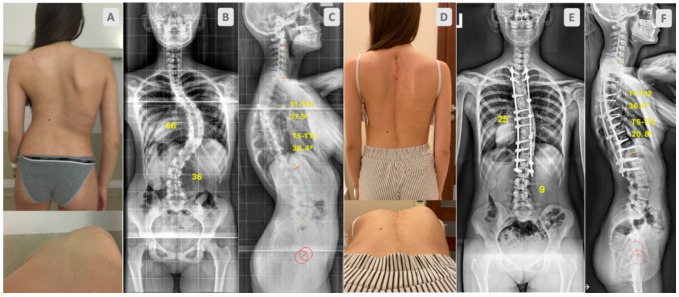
Sixteen-year-old girl with adolescent idiopathic scoliosis. Preoperative clinical pictures (A), standing radiographs, AP (B), lateral (C). Outcome at 2-year follow up after scoliosis correction surgery with DRC only. Clinical pictures (D), standing radiographs, AP (E), lateral (F).
